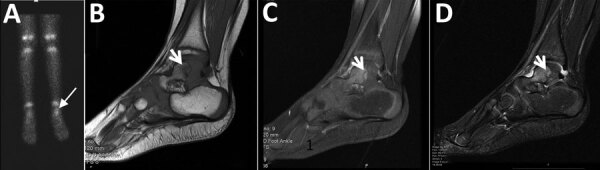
Imaging of the left ankle for a 2-year-old boy (case 2) with Q fever osteoarticular infection, Israel. A) A nuclear bone scan showing uptake in the talus (arrow). B–D) Magnetic resonance imaging sagittal T1 (B), sagittal T1 fat saturation + contrast (C), and sagittal short-TI inversion recovery (D) showing a lesion (white arrows) in the posterior aspect of the talus, noted to be an intramedullary Brodie's abscess in evolution, surrounded by intramedullary edema and accompanied by fluid in the joint.
